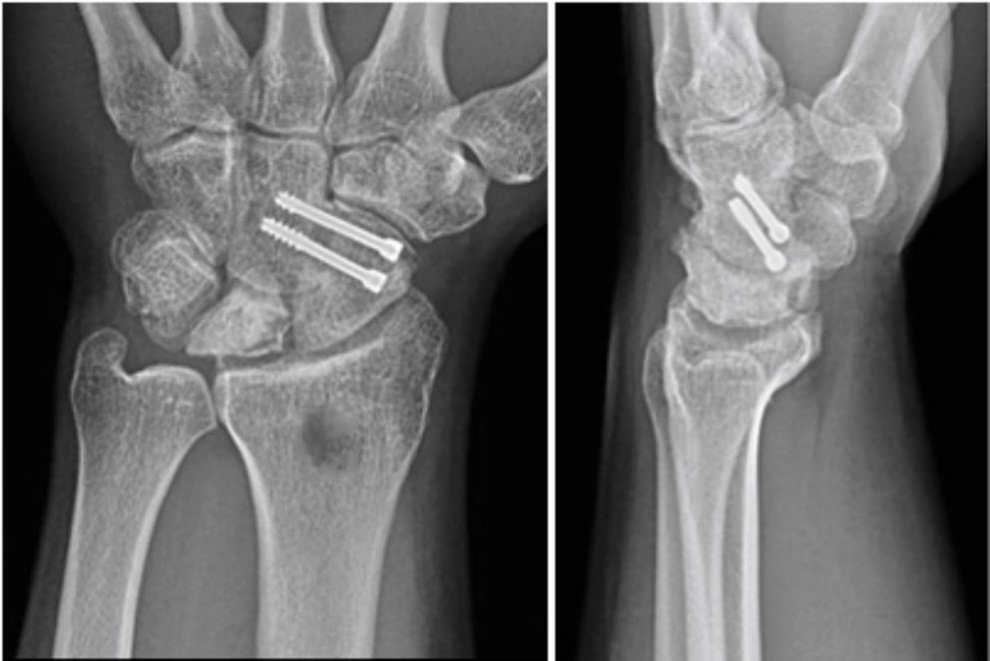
Radiographic posteroanterior and lateral views demonstrating union of the SC arthrodesis stabilized by two 3-mm HCS (Zimmer)
